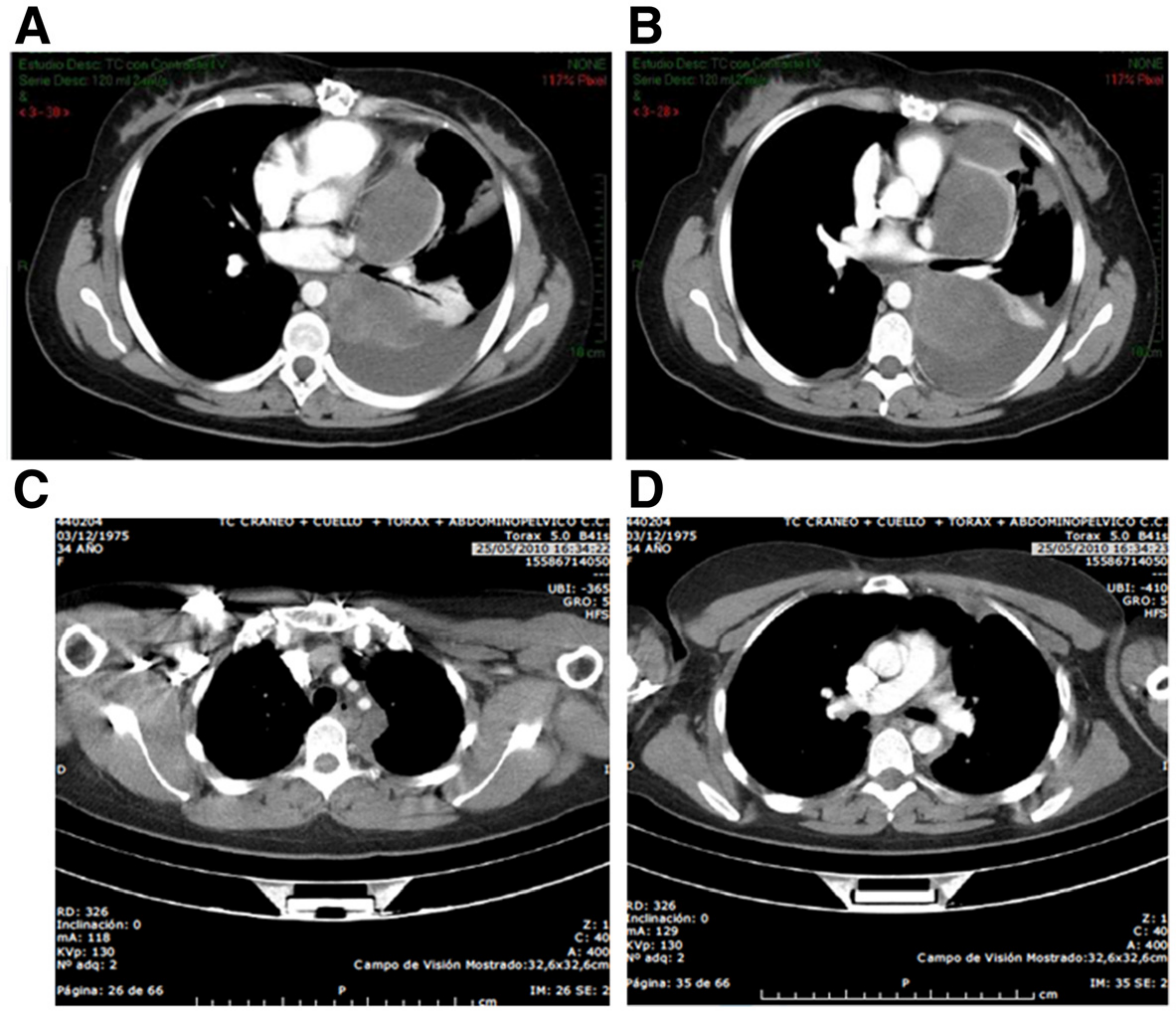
Computed tomography scan of the thoracic region, before (A,B) and after (C,D) treatment with trabectedin (12 cycles).
